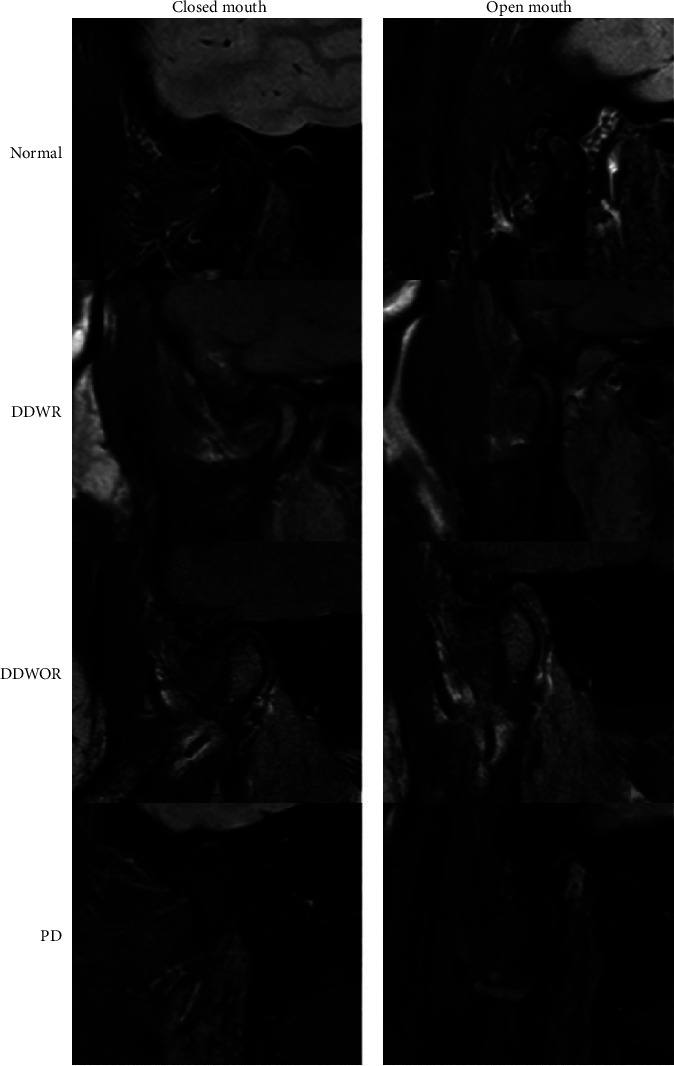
TMJ disc positions obtained from MRI of the closed-mouth and open-mouth positions showed normal DDWR, DDWOR, and PD. DDWR: disc displacement with reduction, DDWOR: disc displacement without reduction, and PD: posterior displacement.
